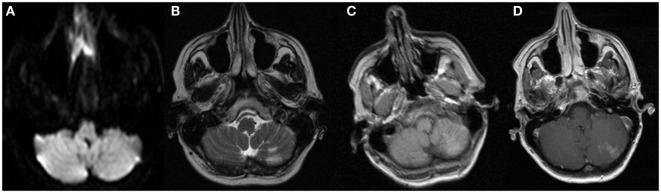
MRI sequences from admission to the OSH 2 days prior to admission at our hospital. These images demonstrate the cerebellar subacute infarction with no restricted diffusion, but contrast enhancement. These images remained unchanged 2 days later on admission to our hospital. (A) DWI, (B) T2, (C) T1 precontrast, and (D) T1 postcontrast.
